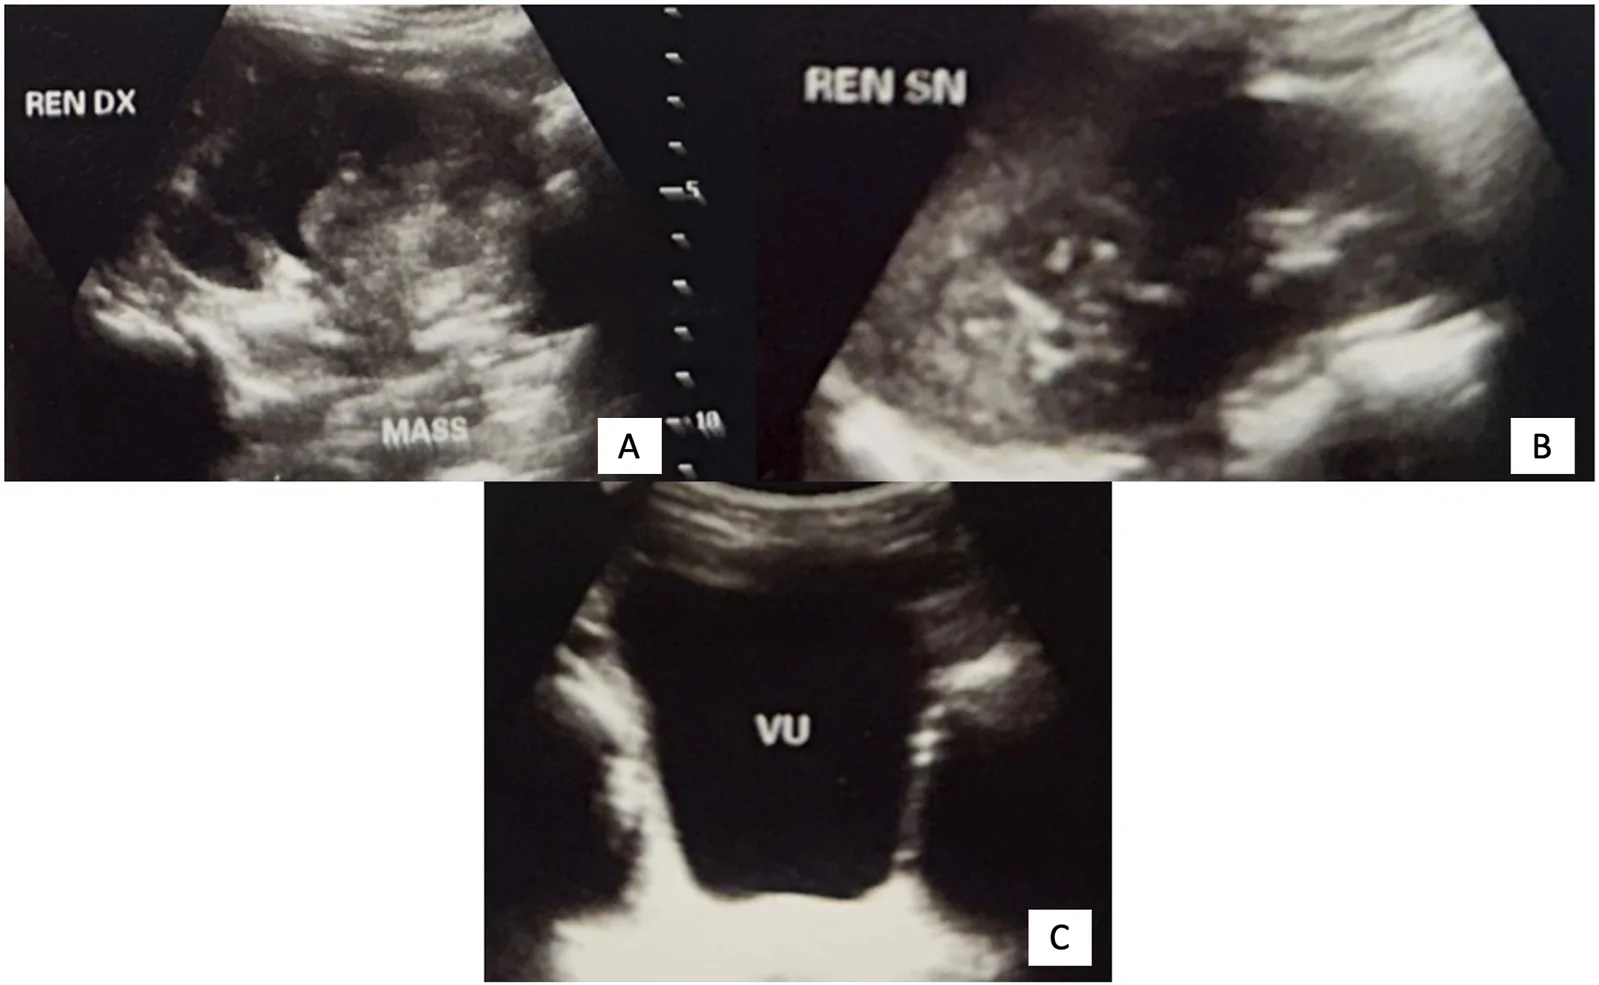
Ultrasonographic examination of right kidney (A), left kidney (B), and bladder (C). This findings showed mass on the right kidney.
